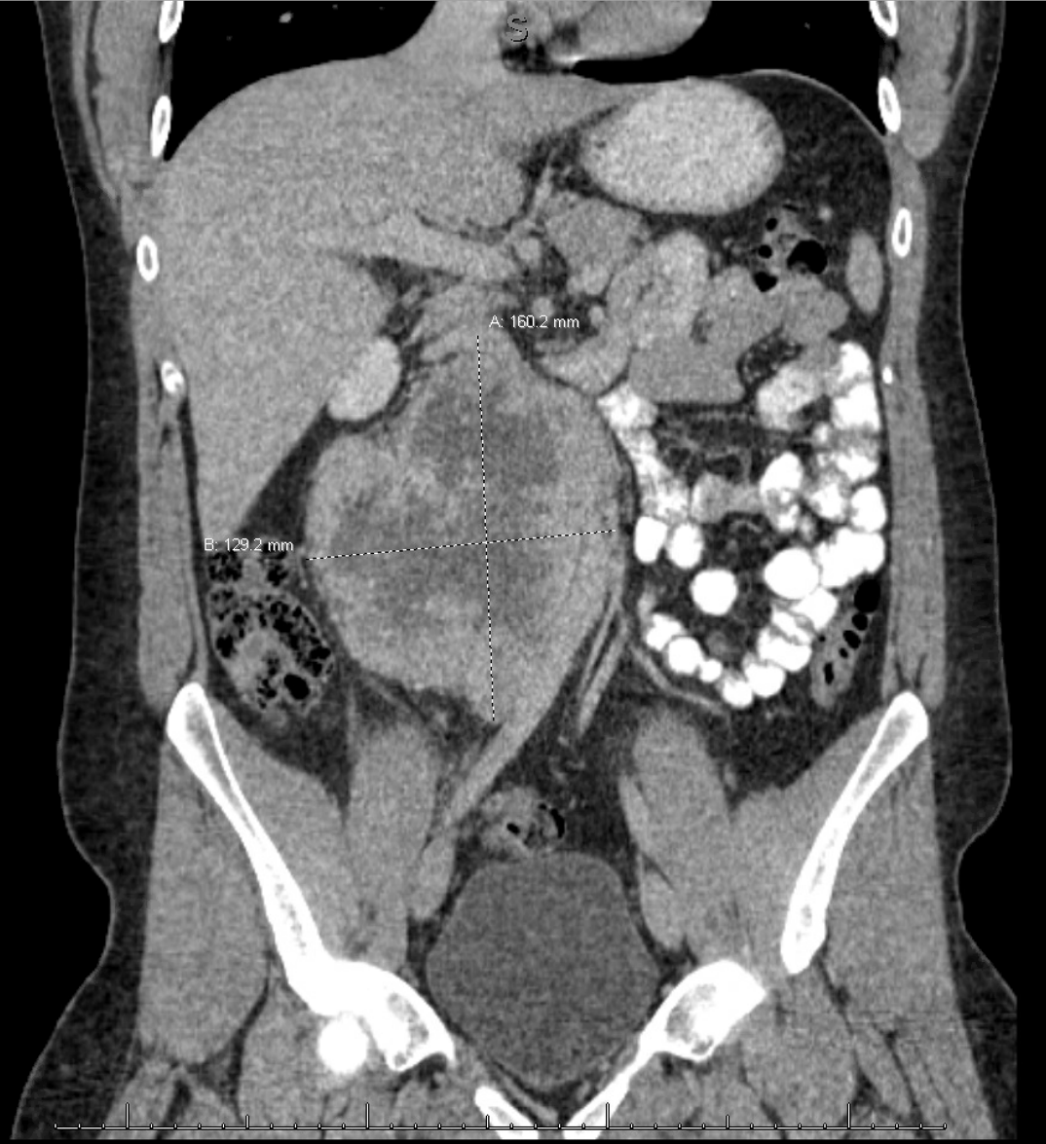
CT abdomen/pelvis with IV contrast – demonstrating 16 x 10 x 13.7 cm retroperitoneal mass encasing and displacing the IVC anteriorly, the ureter medially, and the aorta laterally. The mass extends from the renal vessels to the common iliac vessels.
